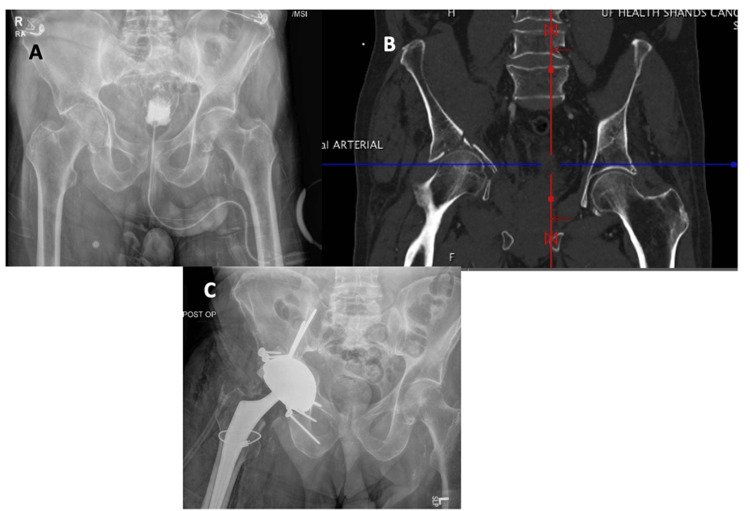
A 72-year-old man with (A) pre-operative AP pelvis showing a right acetabular fracture, (B) pre-operative CT scan showing a right acetabular fracture, and (C) post-operative AP pelvis showing ORIF with acute THA using a metal bimobile acetabular component. THA: Total hip arthroplasty; ORIF: open reduction internal fixation
